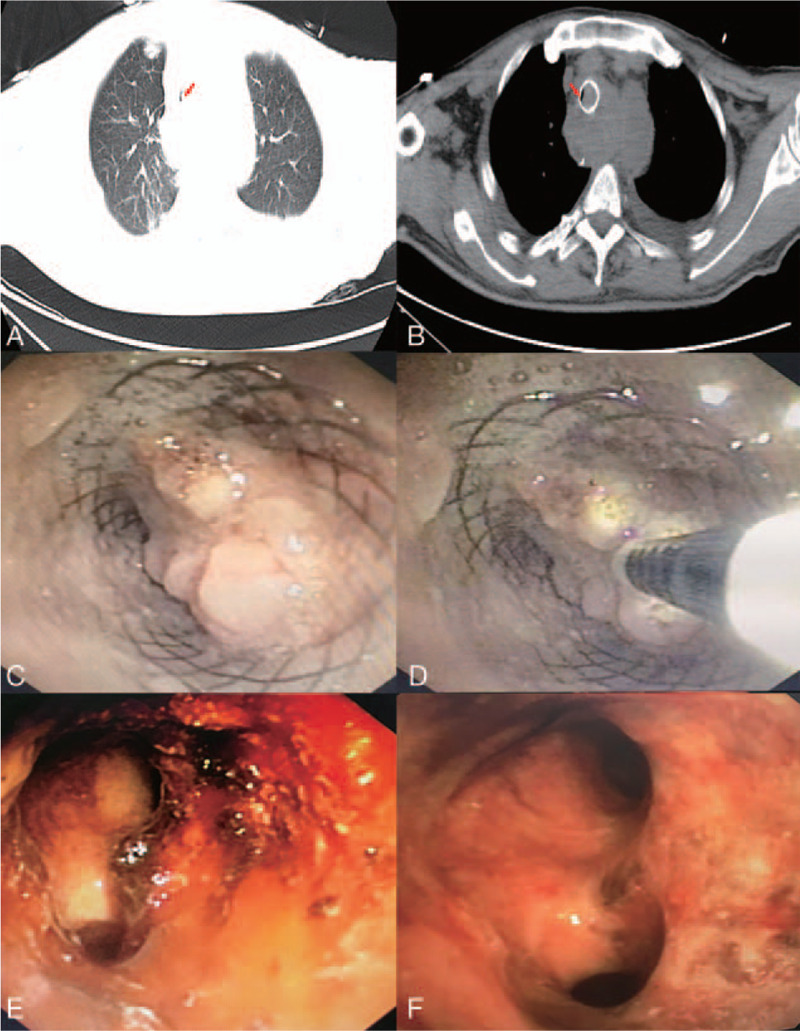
(A and B) Chest computed tomography scan showing tracheal invasion by the esophageal tumor, as well as invasion of the opening of the left and right main bronchi; the tumor tissue blocked the stent and the lumen was severely narrowed (red arrow). (C) Bronchoscopy showing the middle and lower parts of the trachea and slit-like stenosis of the left and right main bronchi, and the highly vascularized tumor. (D) Argon plasma coagulation was used during bronchoscopy-guided intervention therapy. (E and F) The trachea and the left and right main bronchi were recanalized after therapy.
